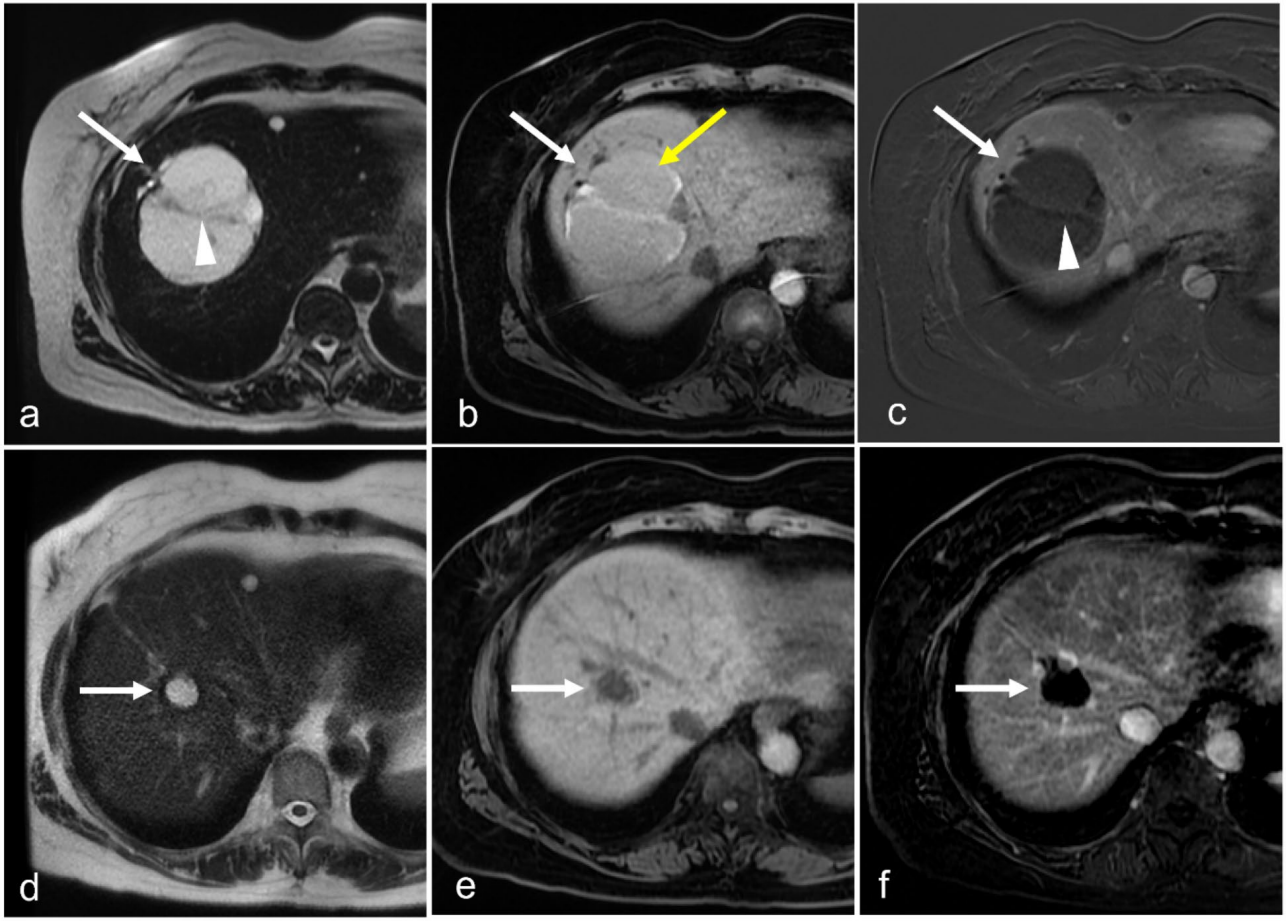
Hemorrhagic cyst in a 68-year-old female patient with history of breast cancer and elevated alkaline phosphatase level. Axial T2-weighted MR image (a) demonstrates a large right hepatic lobe cyst with a thickened-appearing septation (white arrowhead) and upstream biliary ductal dilatation (white arrow). Precontrast axial T1-weighted image (b) demonstrates a T1-hyperintense rim and septa suggesting methemoglobin. Mild T1 hyperintense fluid within the cyst suggests proteinaceous or hemorrhagic contents (yellow arrow). Subtraction postcontrast axial T1-weighted image in the late arterial phase (c) demonstrates minimal enhancement of the wall (arrow) and septa (arrowhead). The patient underwent biopsy, which was nondiagnostic. Because of the presence of biliary ductal dilatation and concern for biliary cystadenoma, surgery was recommended. The patient chose to defer the surgery and observe. Serial imaging every 3 to 6 months demonstrated a decrease in the size of the cyst. The patient’s alkaline phosphatase levels continued to improve. Axial T2-weighted imaging performed 16 months after the initial scan (d) demonstrates a substantial decrease in the cyst size and nearly resolved duct dilatation (white arrow). Precontrast axial T1-weighted image (e) demonstrates resolution of the methemoglobin and proteinaceous or hemorrhagic contents. Subtraction postcontrast axial image in the late arterial phase (f) demonstrates no enhancing septa or nodularity. Overall, the findings were believed to represent resolving hemorrhage within a peribiliary cyst
